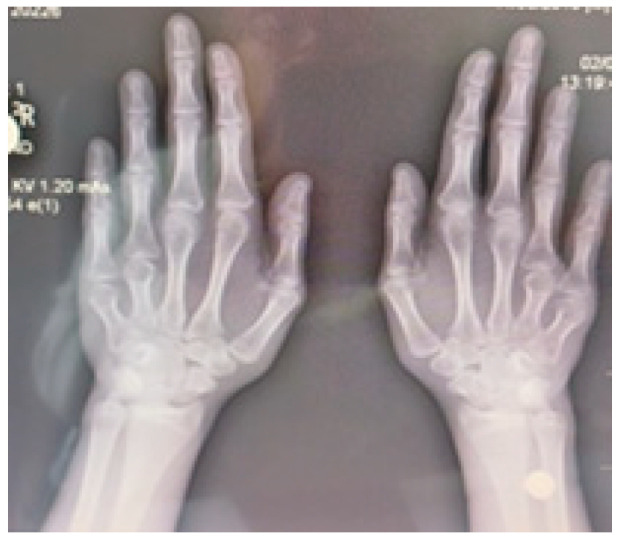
Radiograph of both hands demonstrating bilateral hypoplasia of the fourth and fifth metacarpal bones.
